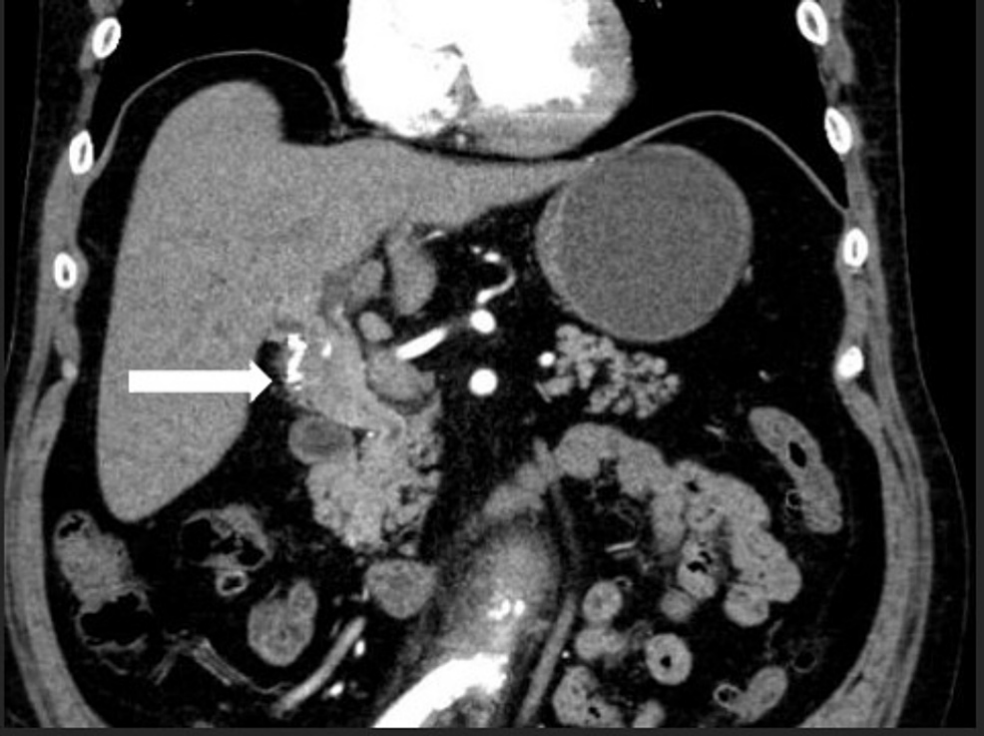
CTA of abdomen and pelvis with IV contrast coronal view White arrow pointing to a 3.1 x 2.8 cm mass compressing the common bile duct. CTA - computed tomography angiogram
